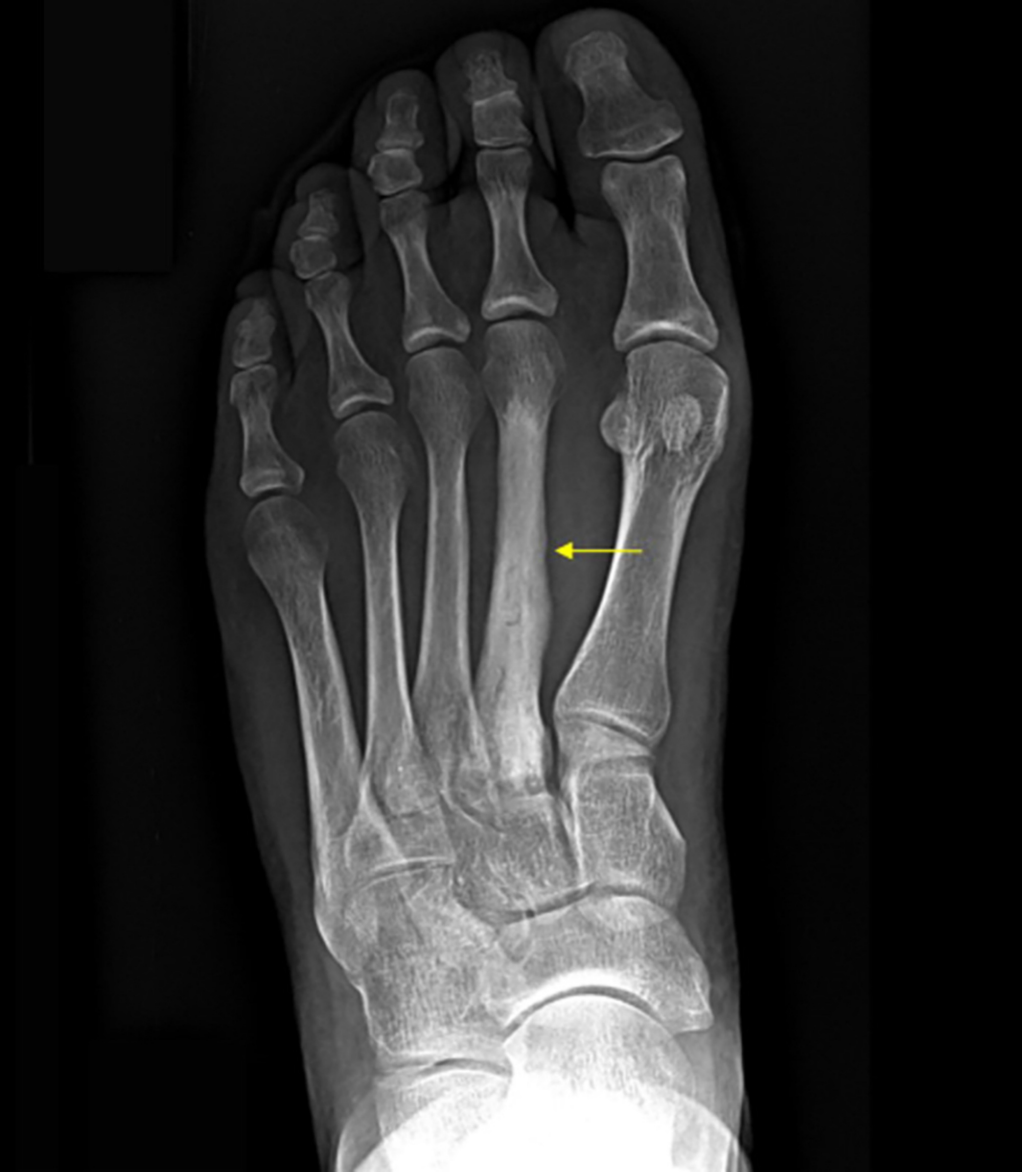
Pre-operative X-ray showing cement in the second metatarsal.
